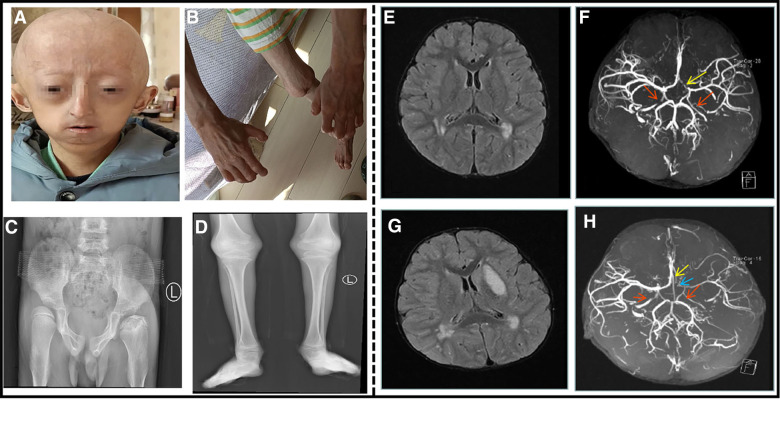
An 8.5-year-old boy patient. (A) The child showed distinctive facial features, with prominent forehead, baldness, exposed scalp veins, long and narrow nose, thin upper lip, and micrognathia. (B) The child exhibited flexion deformity of the distal knuckle joints of both the fingers, abduction of the bilateral wrist. (C) Anteroposterior pelvis X-ray showed bilateral hip dysplasia, bilateral femoral heads laterally positioned and left femoral head aseptic necrosis. (D) Long bones with thin diaphysis, widened metaphysis, and decreased bone density. (E) Cranial MRI at the time of onset: multiple abnormal signal shadows in the lateral ventricle. (F) Cranial MRA at the time of onset: bilateral internal carotid artery stenosis. The red arrow indicates internal carotid artery stenosis, and the yellow arrow indicates left anterior cerebral artery stenosis. (G) After the 3-month review, cranial MRI: multiple abnormal signal shadows in the lateral ventricle, and the left basal Nodal lesions. (H) After the 3-month review, cranial MRA: his left internal carotid artery, left middle cerebral artery and left cerebral artery had stenosis. The red arrow indicates internal carotid artery stenosis, the blue arrow indicates left middle cerebral artery stenosis, and the yellow arrow indicates left anterior cerebral artery stenosis.
